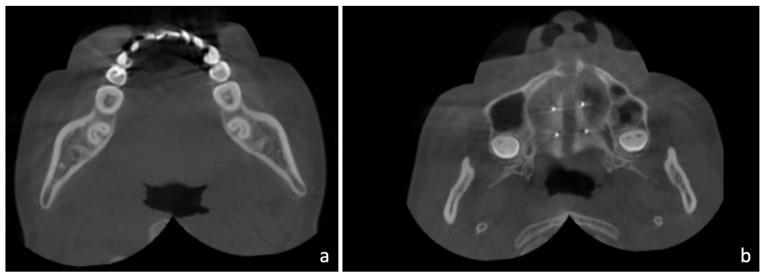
Axial sections of the cone beam computerized tomography (CBCT). (a) Distal roots of second molars were close to the lingual cortex of the alveolar process, which means the spaces for posterior distalization are minimal. (b) Disengagement of the pyramidal process from the pterygoid notch was noted in the left pterygopalatine suture. The opening is present between the pyramidal process and the lateral pterygoid plate.
